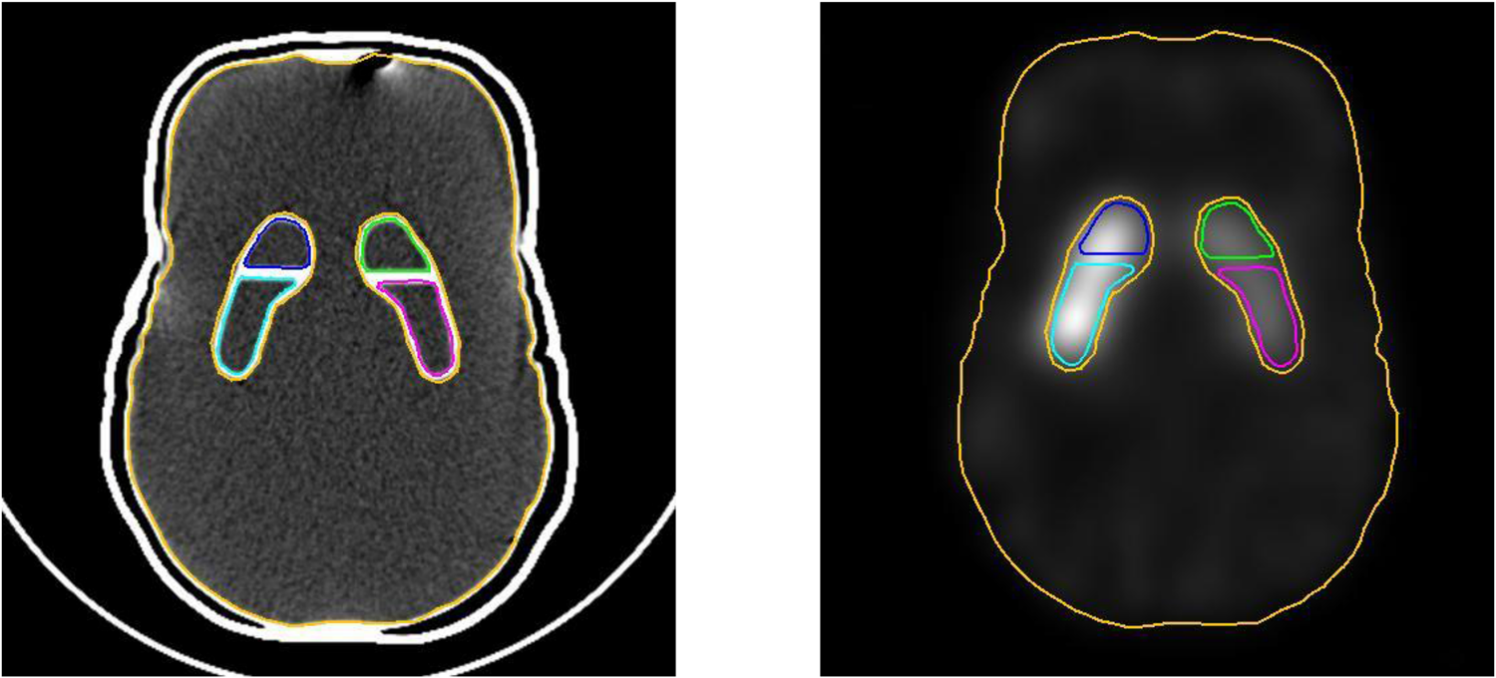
The establishment of the volume of interests (VOIs). The left image shows the establishment of VOIs of the caudate, putamen, and the whole brain as background (BG) regions on computed tomography. The right image shows the VOIs of each region on single‐photon emission computed tomography
